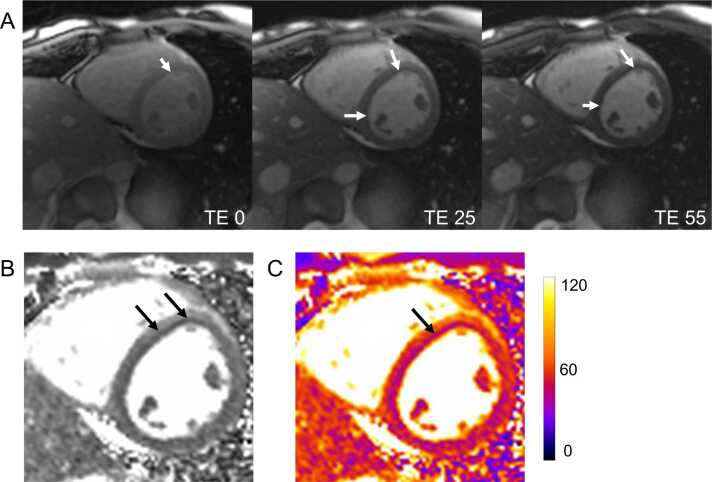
Gibb’s ringing. (A) T2-weighted source images, acquired in end-diastole showing a subendocardial septal dark band (white arrows), indicative of Gibbs ringing, which is also present on the final T2 map (B) and color-coded T2 map (C). Gibbs ringing commonly occurs at sharp interfaces between tissues with high differences in signal intensity, as observed here at the border between blood and myocardium. The dark band in myocardium is more prominent when the change in brightness at the blood and myocardium border is greater, which is typical for Gibbs ringing. Note that the Gibbs ringing is missing on the RV side of the septum which might be due to residual motion. *RV* right ventricular, *TE* echo time
